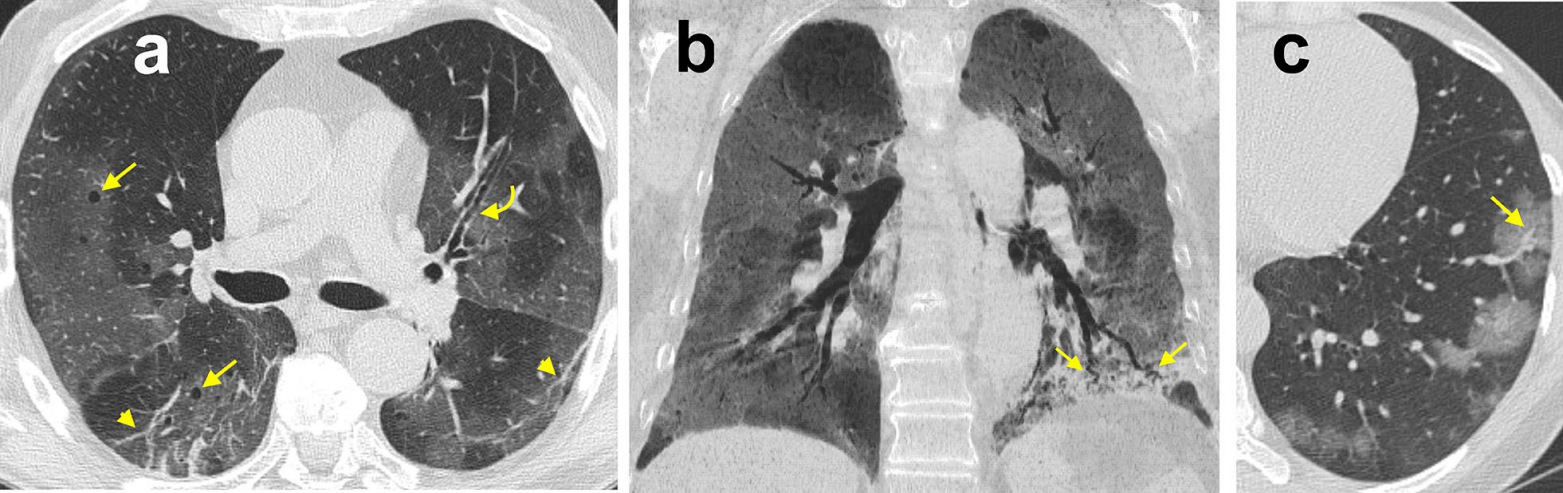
Ancillary chest CT imaging findings in COVID-19 pneumonia. a High-resolution CT image of a 73-year-old male COVID-19 patient presenting fever with dry cough for 5 days shows bilateral, multifocal, peripheral areas of ground-glass opacity (GGO) with mosaic appearance, along with superimposed fibrous stripes in the lower lobes (arrowheads), bronchial wall thickening (curved arrow) and bubble-like air-containing spaces (straight arrows). b 8-mm Min-IP coronal CT image of a 73-year-old female COVID-19 patient presenting fever for 5 days shows bilateral, multifocal, peripheral GGO, along with consolidation in the left lower lobe presenting with air bronchogram and bronchus deformation (arrows). c High-resolution CT image of the same patient as inset (a) shows dilatation of pulmonary vessels within one of the multiple GGO in the left lower lobe (arrow)
